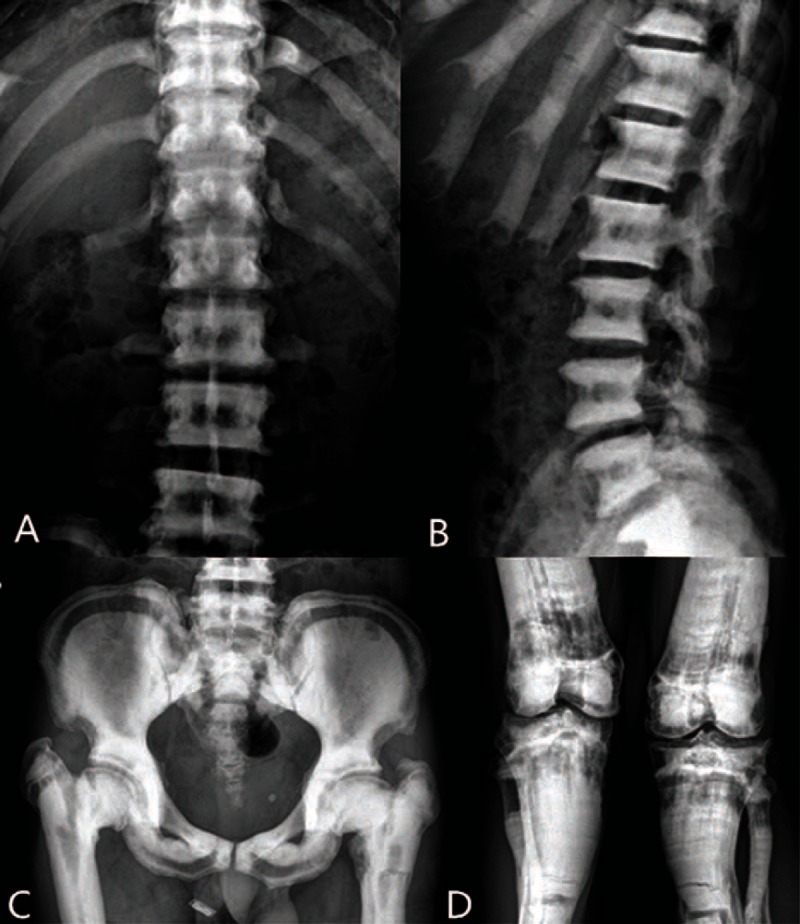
X-ray images of patient's spine, pelvic and both knees.
